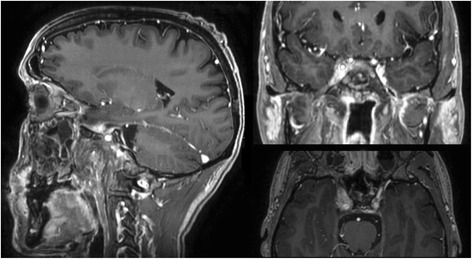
T1-weighted MRI in December of 2016 showing excellent subtotal tumor response following re-re-irradiation in sagittal, coronal and axial plane
